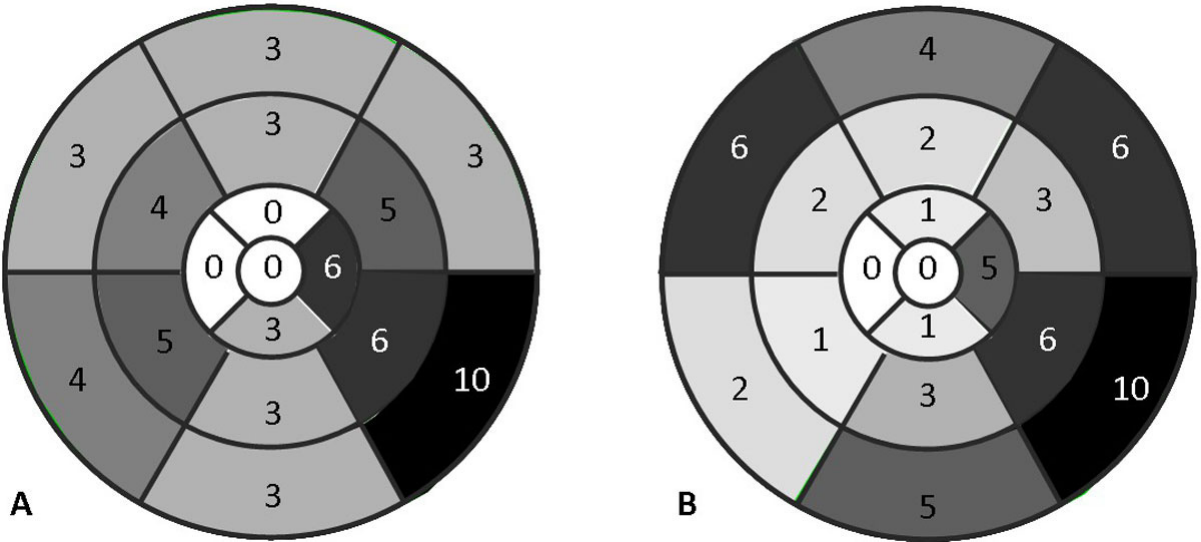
LV distribution of LGE (A) and significant myocardial FDG uptake (B) for all 30 patients. By both modalities, cardiac sarcoidosis predominantly involves the lateral wall with relative sparing of the distal anterior wall, distal septum and apex.
